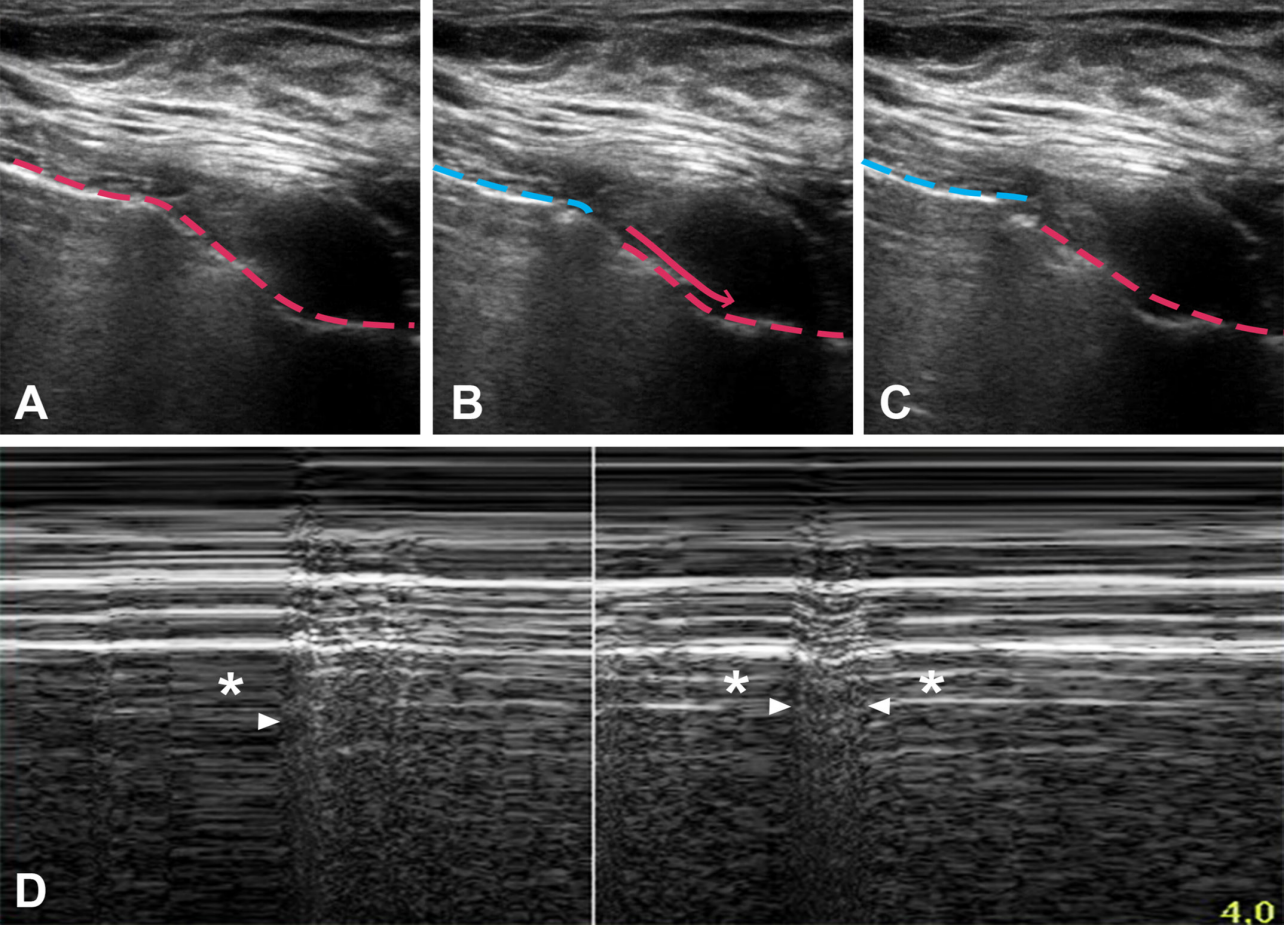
Typical lung ultrasound findings in patients with bullous emphysema: Bulla‐point and barcode sign. (A–C) Bulla‐point shown in B‐mode. Pictures were made at different points of the respiratory cycle: Rest (A), beginning of inspiration (B), end of inspiration (C). The pleuropulmonary interface is represented by a dotted line. During inspiration, a discontinuity occurs in the pleural line, as normal pleural sliding is observed in the caudal part of the image (red dotted line with arrow) while the cranial part shows comparatively much decreased pleural sliding (blue dotted line). A video version is presented in the Supporting Information (Video S1). (D) Barcode shown in TM mode (arrowheads). The asterisk indicates the beginning of a voluntary breath holding manoeuvre.
